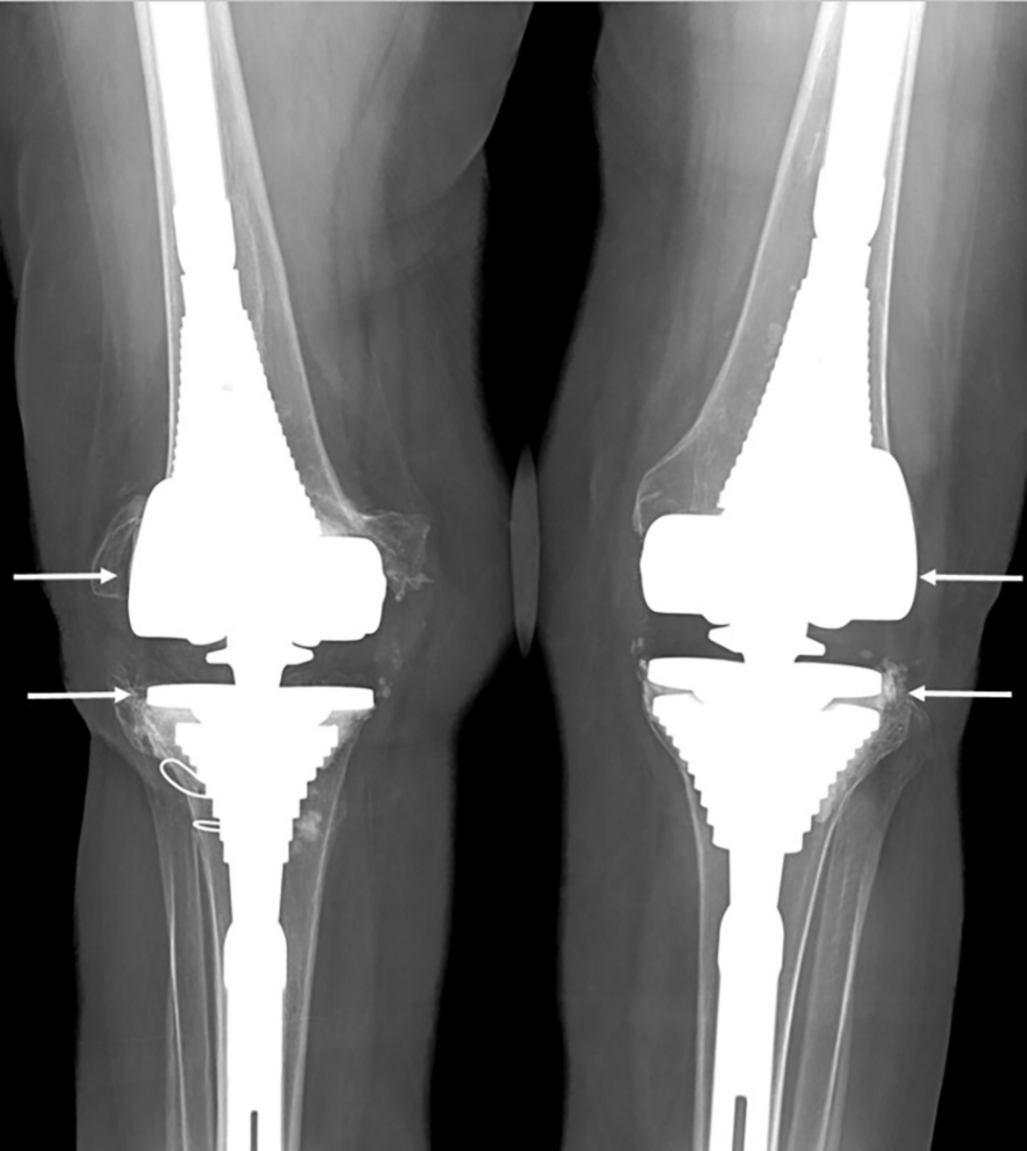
Postoperative X-ray showing intact hardware following bilateral revision total knee arthroplasty for instability
